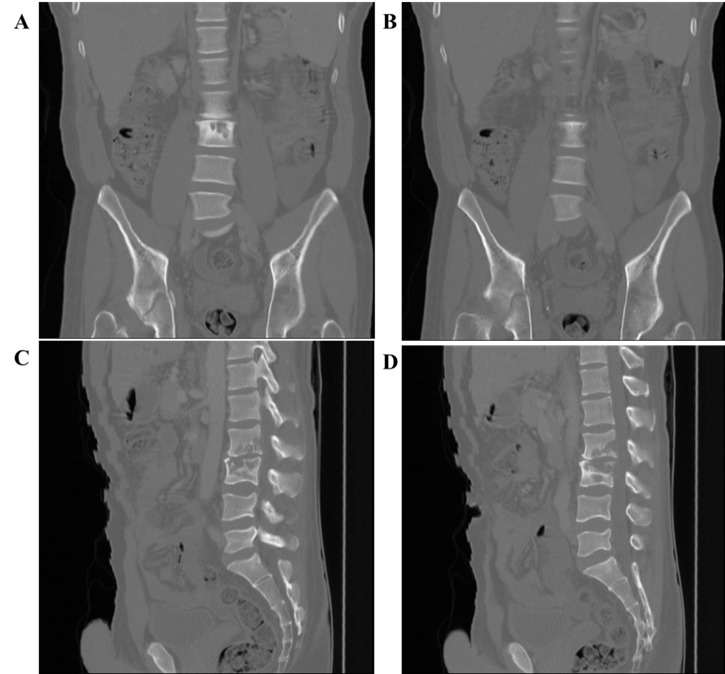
CT scan lumbar spine and pelvis AP and lateral views
